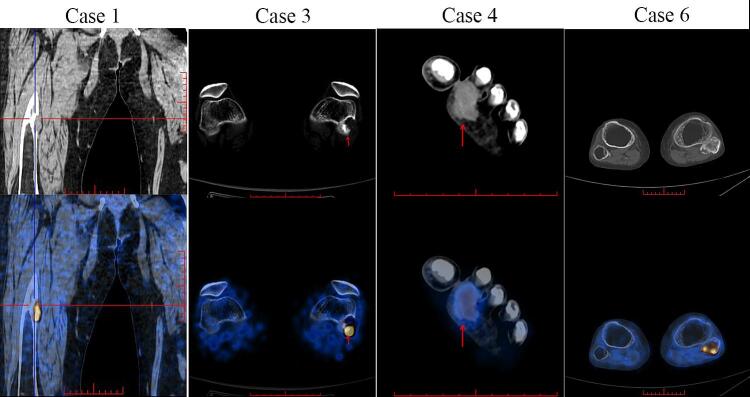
Positron Emission Tomography-Computed Tomography(PET/CT). In Case 1, 3, and 4, 68Ga-DOTATATE PET/CT revealed radiotracer-avid lesions located in the mid-right femur, lateral condyle of the left distal femur, and soft tissue of the left foot, with maximum standardized uptake values (SUVmax) of 17.8, 6.9, and 4.9, respectively. In Case 6, an 18F-FDG PET scan showed a radiotracer-avid lesion at the left fibular head, with an SUVmax of 4.7. For Case 2, imaging was performed at an outside hospital, and image data were not available. Case 5 did not undergo PET imaging.
